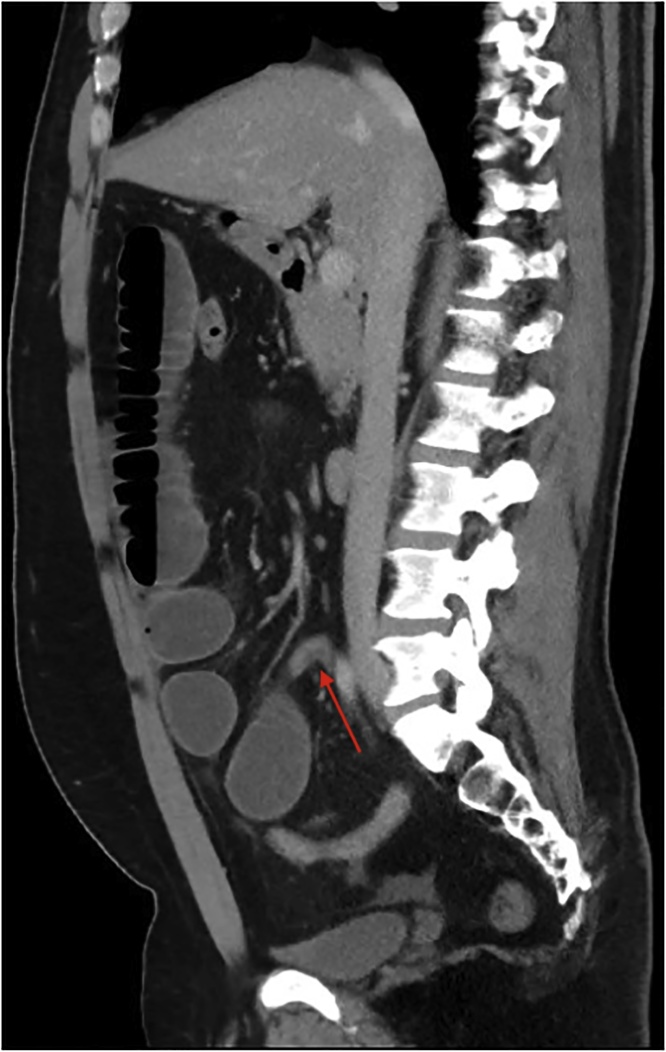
Sagittal view of the CT abdomen and pelvis showing transition point of the SBO with the angulation of the small bowel suggesting a kink.
